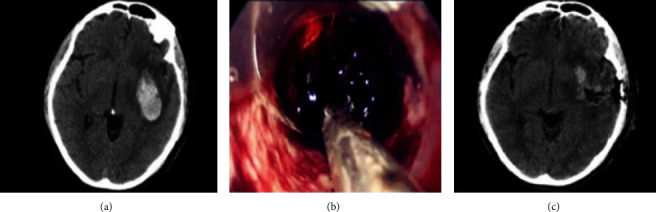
A 54-year-old male patient with hypertensive cerebral hemorrhage in the posterior basal ganglia region: (a) preoperative CT; (b) intraoperative CT; (c) postoperative CT.
